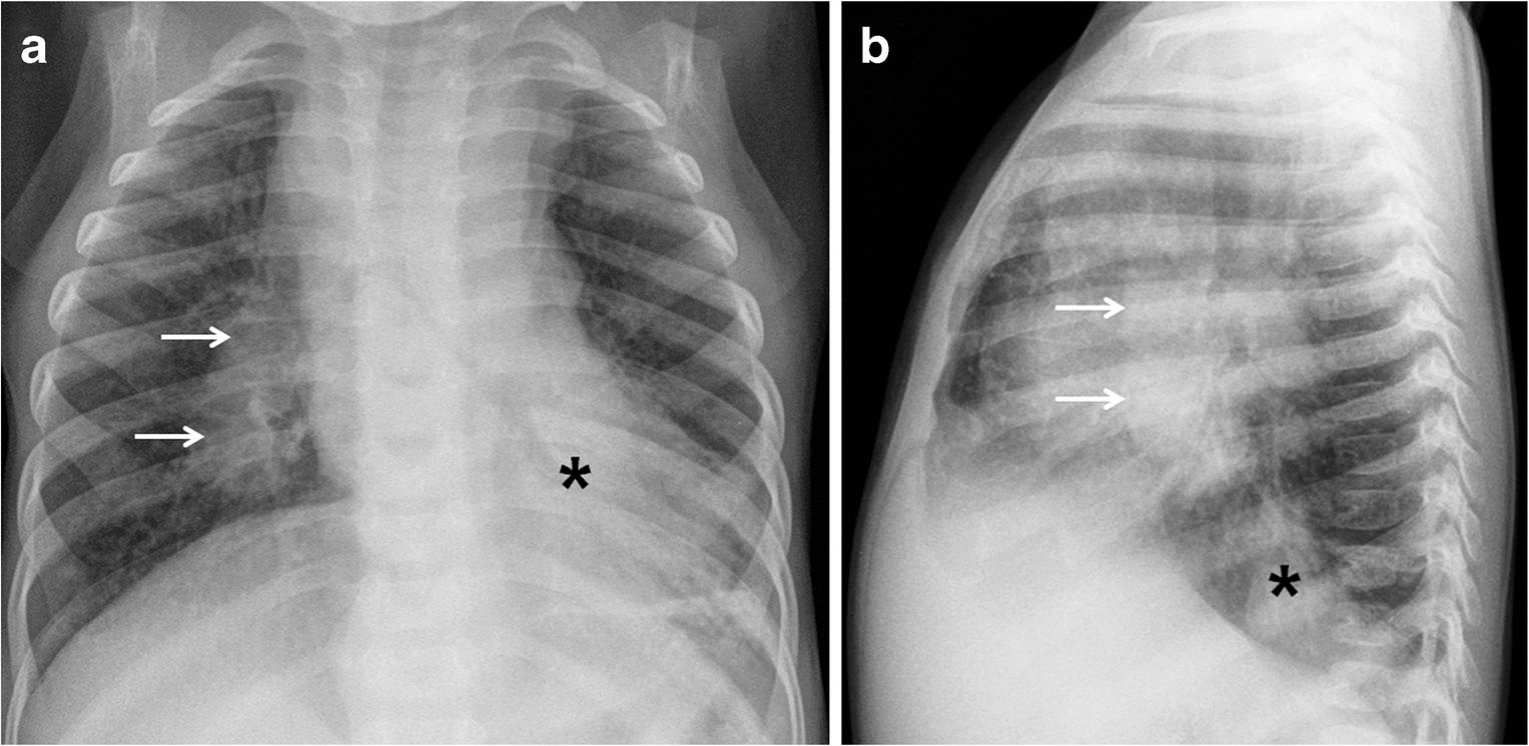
Chest radiographs in a 1-year-old boy with primary tuberculous disease and lymphadenopathy. a, b Anteroposterior (a) and lateral (b) views show hilar lymphadenopathy (*arrows*) on the right without ipsilateral lung abnormality. A left retrocardiac opacity (*asterisk*) is noted
